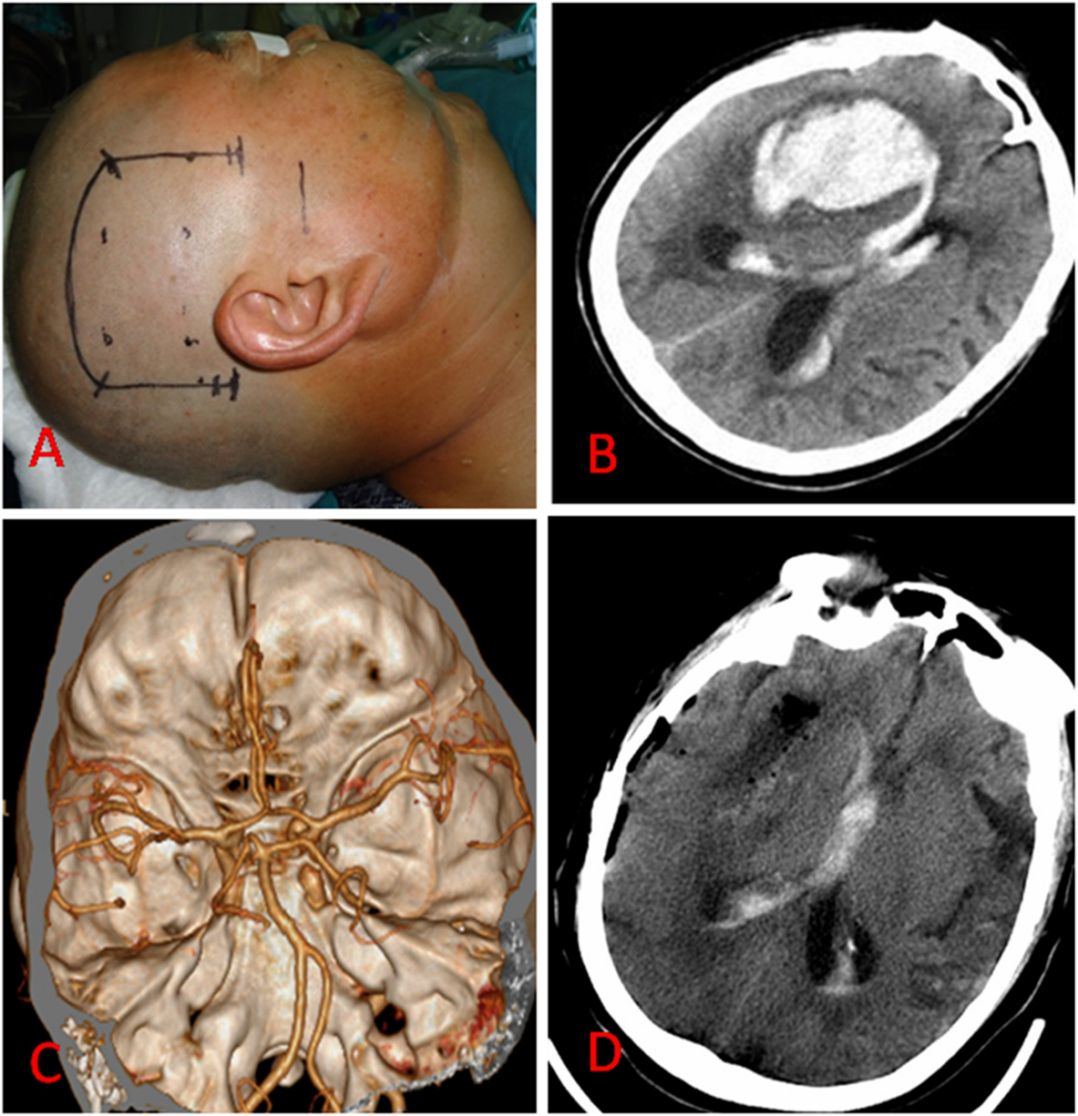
The basal ganglia hematoma was evacuated by Transcortical-transtemporal approach. A: A horseshoe shape incision line for the parietal-temporal craniotomy; B: The CT scan demonstrated a large hematoma within the right basal ganglia of the head and intraventricular hemorrhage, when the patient was admission to neurosurgery department; C: both of aneurysm and AVM were found on CTA; D: CT scan showed the hematoma within the basal ganglia except of the intriventriclar hemorrhage, was totally removed on postoperative day 2.
